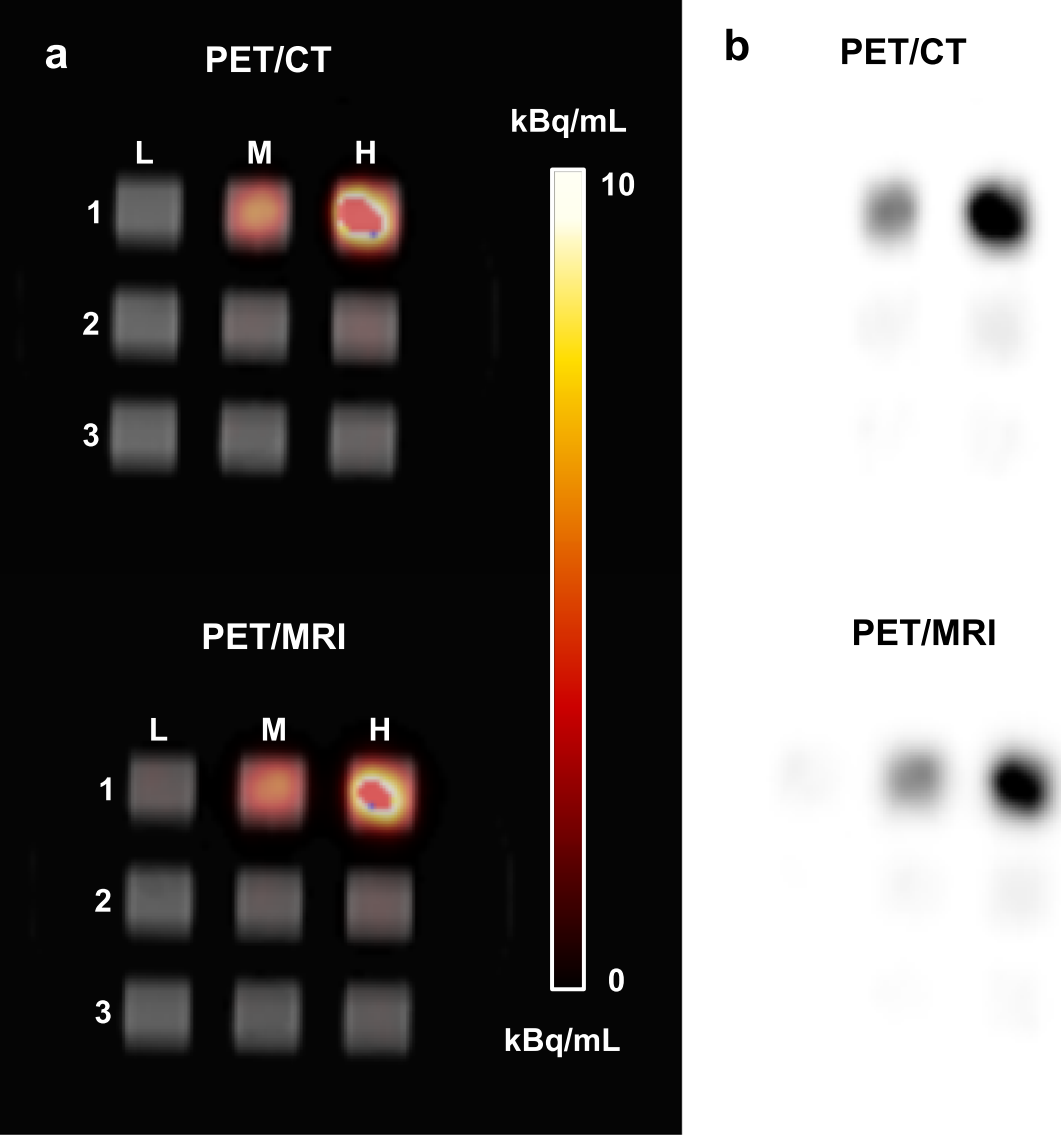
PET images of 3 × 3 cubic-well plate containing ^89^Zr-labeled Jurkat cells suspended in Geltrex™ matrix in a 1-cm^3^ cube acquired using PET/CT and PET/MRI together with their a corresponding co-registered CT image and b in greyscale. Cell numbers and specific activities per well (kBq/10^6^ cells; indicated in parenthesis) were as follows: L1: 1.07 × 10^6^ (0.67). L2: 1.50 × 10^5^ (0.42). L3: 6.70 × 10^4^ (0.14). M1: 8.20 × 10^5^ (7.23). M2: 3.25 × 10^4^ (15.41). M3: 3.61 × 10^4^ (6.61). H1: 3.99 × 10^5^ (30.63). H2: 1.21 × 10^5^ (8.47). H3: 6.20 × 10^4^ (6.60)
